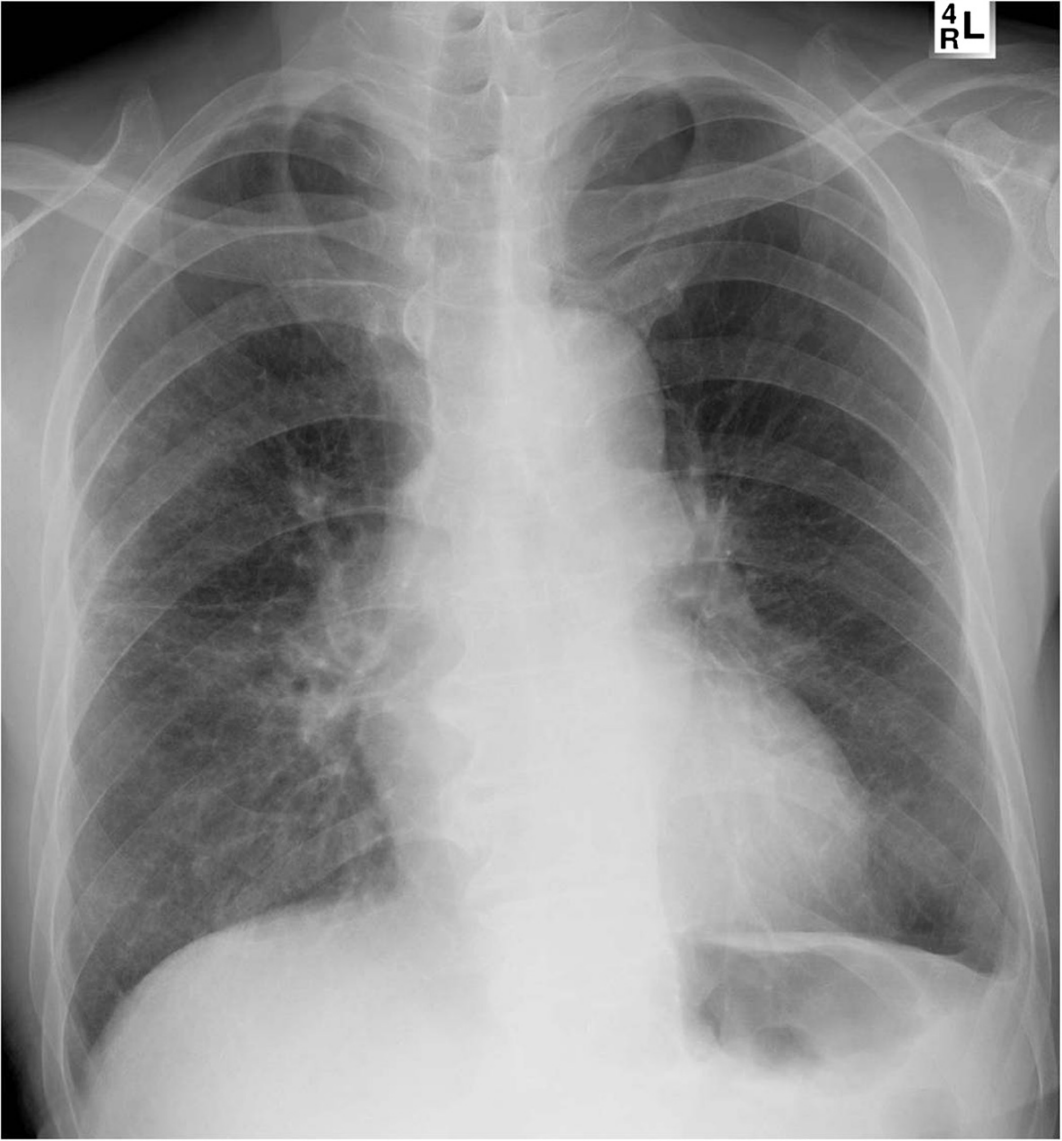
Postoperative chest x-ray. Abnormal air shadows observed preoperatively were not seen.
